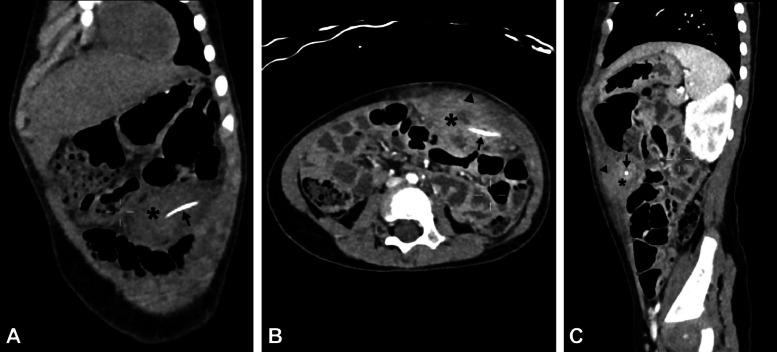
Coronal (A), axial (B), and sagittal (C) abdominal computed tomography images show at the left mid-abdominal region, a linear hyperdense foreign object (arrow), approximately 2.5 cm in length, is visibly lodged within the left intra-abdominal cavity (asterisk), characterized by heterogeneity. This cavity presents as a substantial localized collection, measuring 5.3 × 3.2 × 7.0 cm (craniocaudal × anteroposterior × transverse) in dimensions closely situated adjacent to the anterior abdominal wall (arrowheads).
